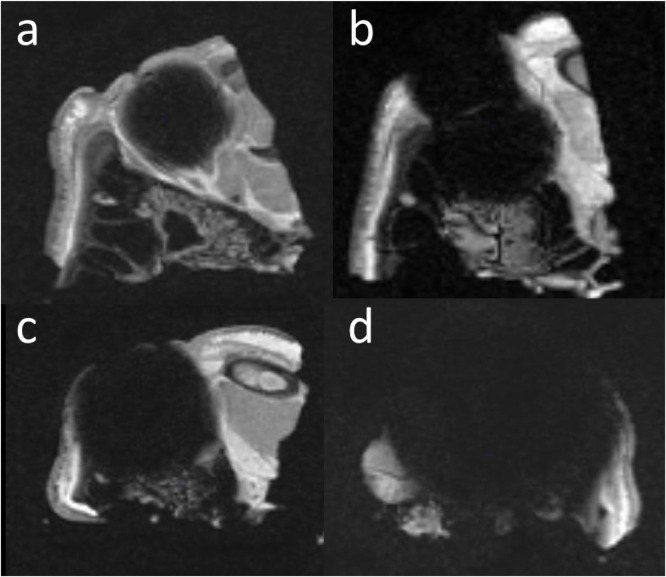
Examples of magnetic susceptibility artifacts on MRI. a: 1-mm ferromagnetic foreign body; b: two 1-mm ferromagnetic foreign bodies; c: 2 mm ferromagnetic foreign body; d: 4 mm ferromagnetic foreign body.
